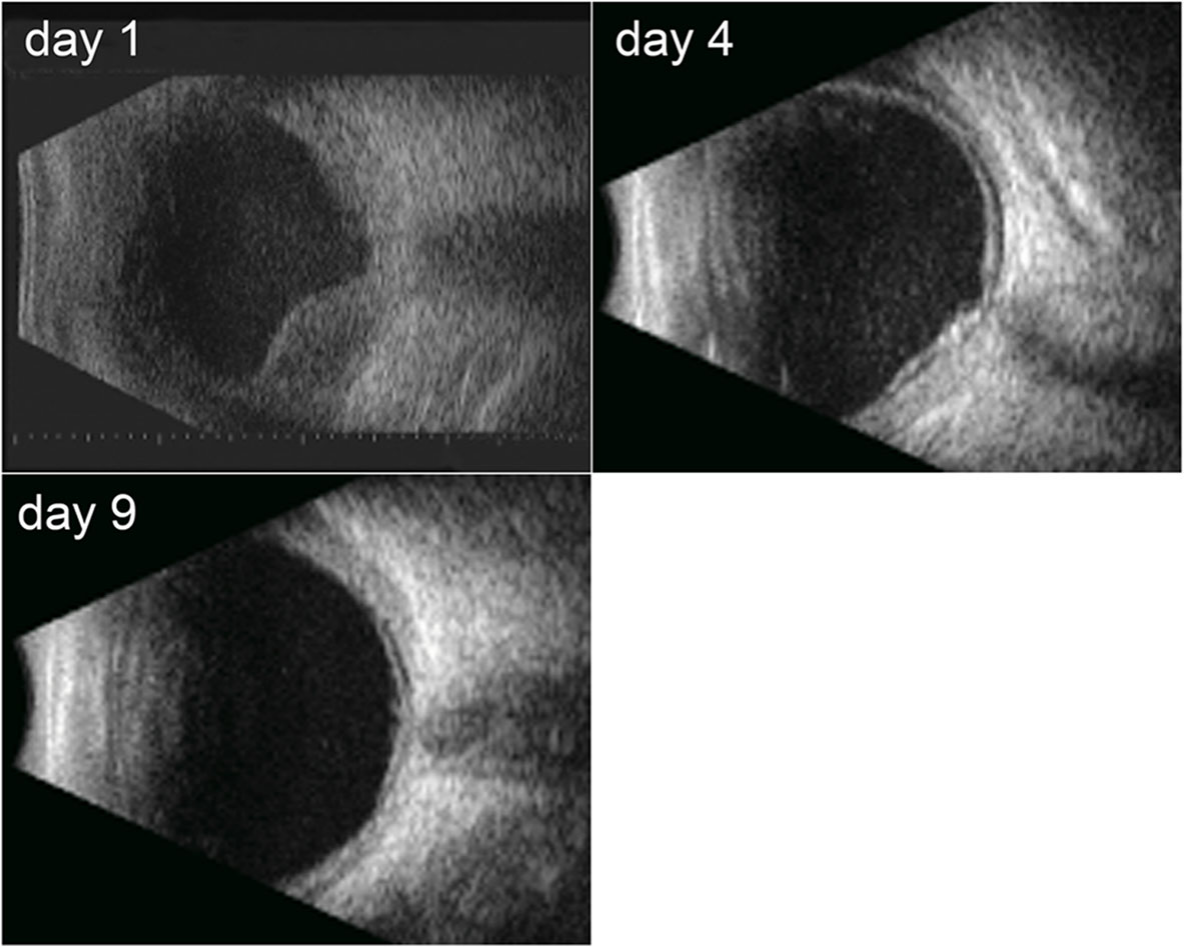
B-scan ultrasound of the right eye showing vitreous opacities and an extensive retinal detachment with sub-retinal exudates on postoperative day 1 (the upper left); slight vitreous opacities with shallow retinal detachment on postoperative day 4 (the upper right); the retina reattached except localized shallow retinal detachment on postoperative day 9 (the bottom left)
